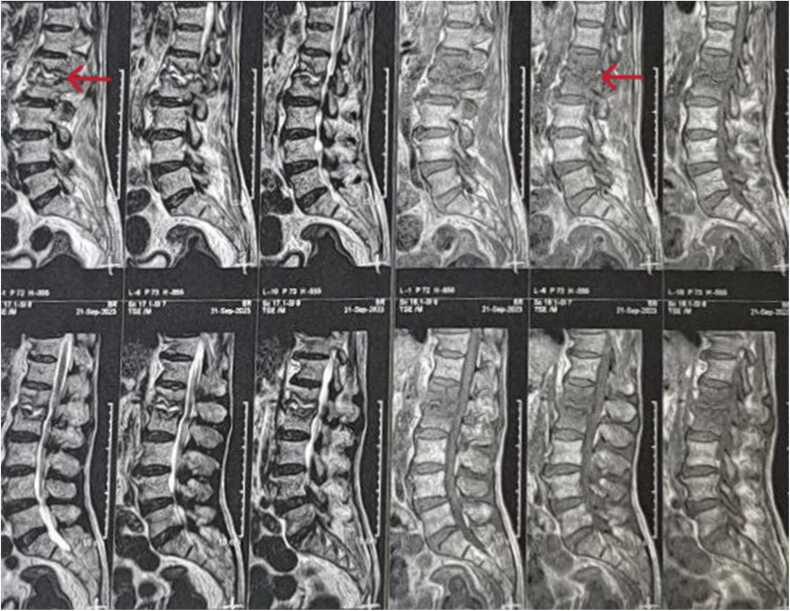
Lumbosacral MRI: disc height loss with sub endplate marrow edema and erosion at L1-L2 level mostly in favor of spondylodiscitis.
